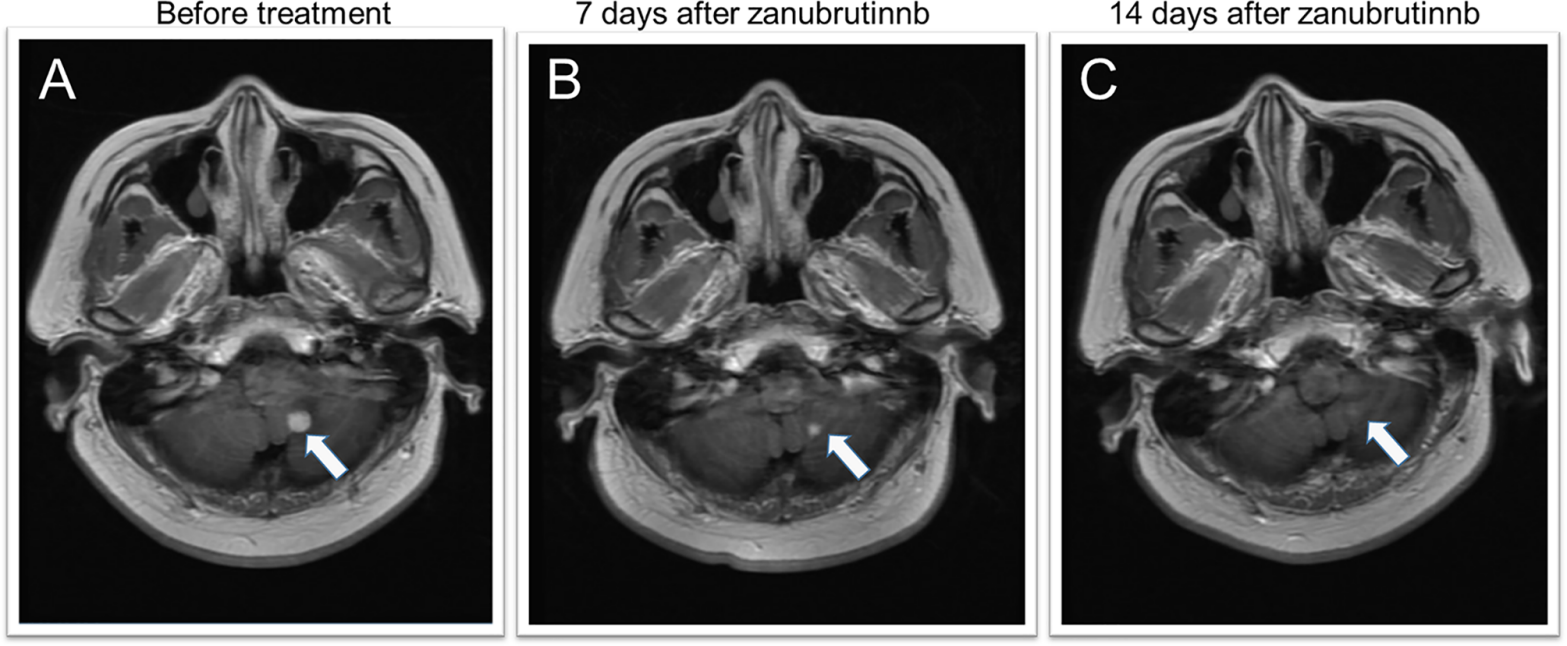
MRI features of a secondary CNSL case during zanubrutinib monotherapy. (A) MRI depicts a homogeneously contrast-enhanced mass in the left cerebellum before zanubrutinib administration. (B, C) 7 and 14 days after zanubrutinib, the mass shrank and disappeared.
